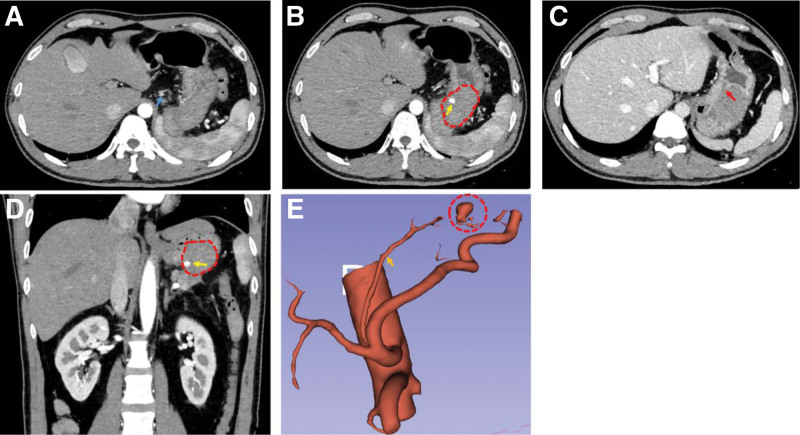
Preoperative enhanced CT of the upper abdomen. (A, B) Arterial phase in transverse view showing the left gastric artery (blue arrow), arterial aneurysm within the pseudocyst (yellow arrow), pancreatic pseudocyst (dotted circle). (C) Portal venous phase in transverse view showing discontinuity of the gastric wall (red arrow). (D) Arterial phase in coronal view showing the arterial aneurysm within the pseudocyst (yellow arrow) and the pancreatic pseudocyst (dotted circle). (E) Three-dimensional reconstruction showing the arterial aneurysm within the pseudocyst (dotted circle). CT = computed tomography.
